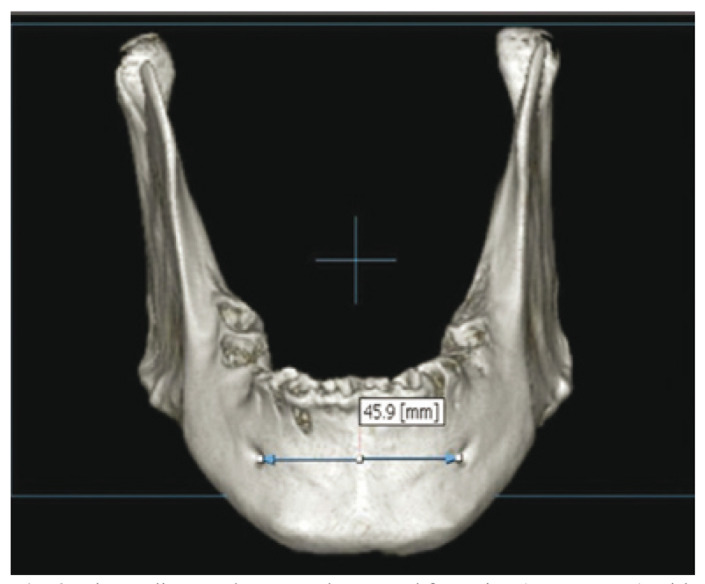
Linear distance between the mental foramina (Ment-Ment) with CBCT.
